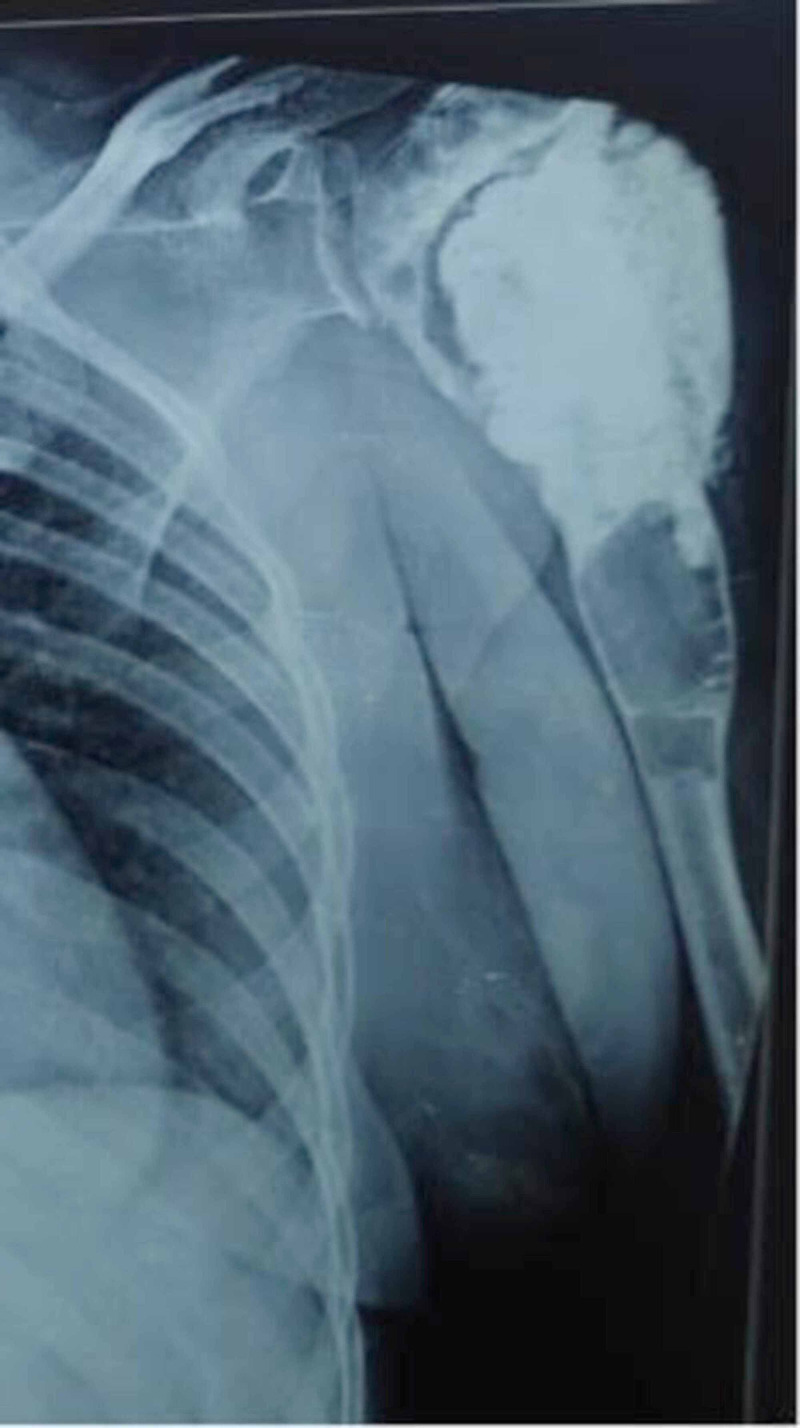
X-ray of the left shoulder showing bone cement separated by a radiolucent zone and osteolytic expansion of the diaphysis below suggestive of third recurrence (2018)
